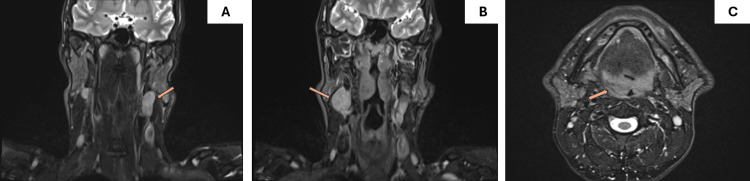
T2-weighted MRI scan without contrast enhancement. A) Coronal plane: Enlargement of left cervical lymph nodes, the largest of which measures 3.3 × 2.2 × 2.6 cm (arrow). B) Coronal plane: Enlargement of right cervical lymph nodes, the largest of which measured 3.8 × 2.2 × 1.9 cm (arrow). C) Axial plane: Asymmetric enlargement of the right palatine tonsil (arrow).
